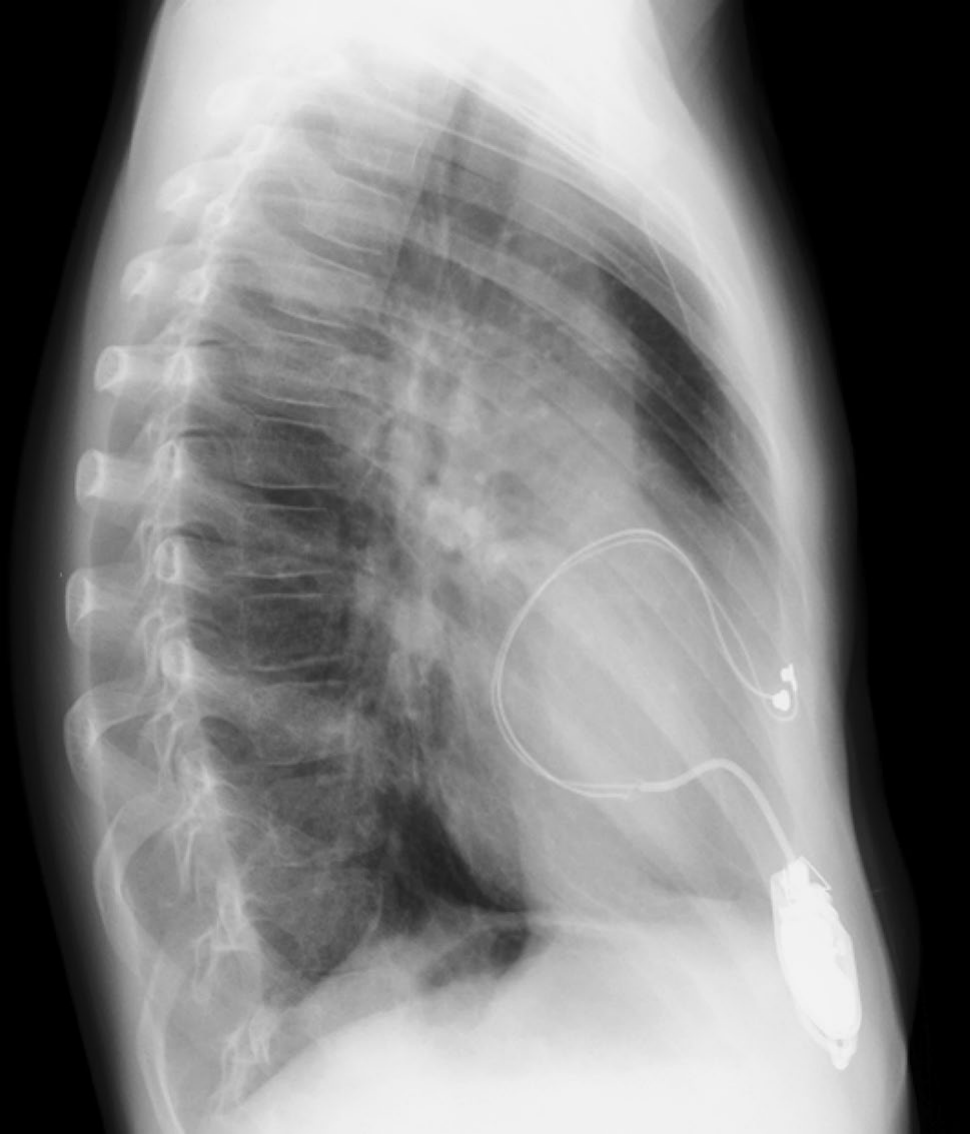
Lateral view of the X-ray at 8 years of age when we noticed the cardiac strangulation
